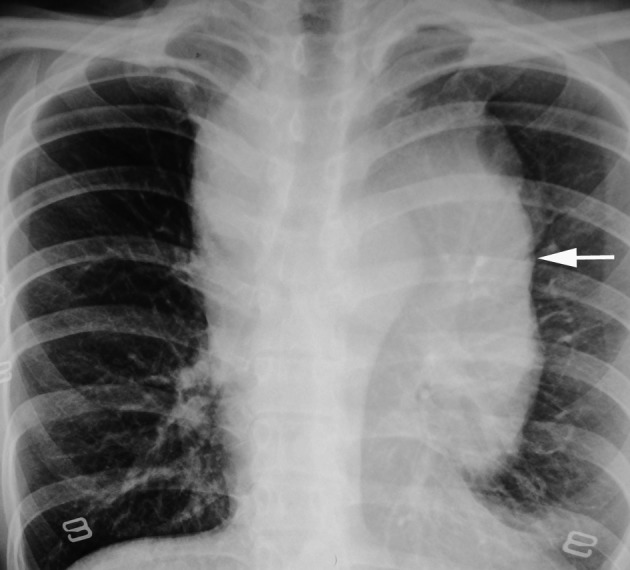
Chest radiograph showing the projecting abnormal giant upper mediastinal shadow (arrow).
